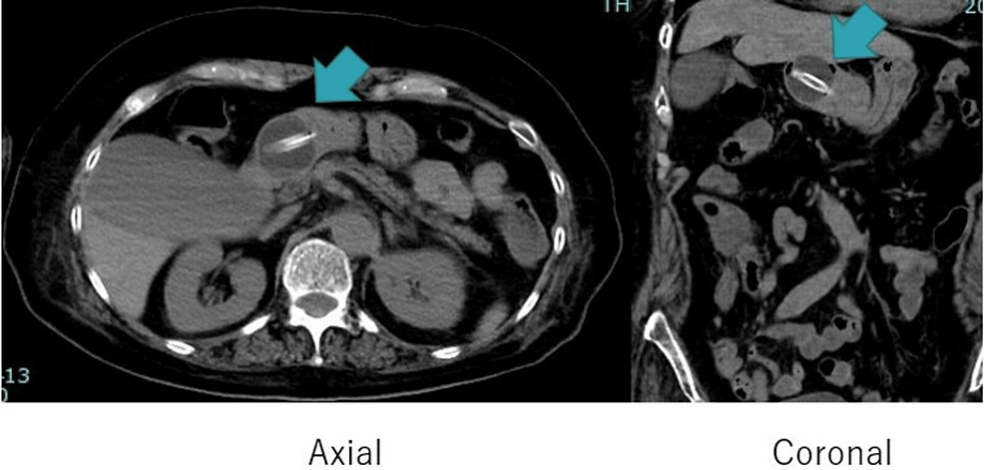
CT image at the time of arrival The tip of the percutaneous endoscopic gastrostomy tube has strayed into the duodenal bulb.
